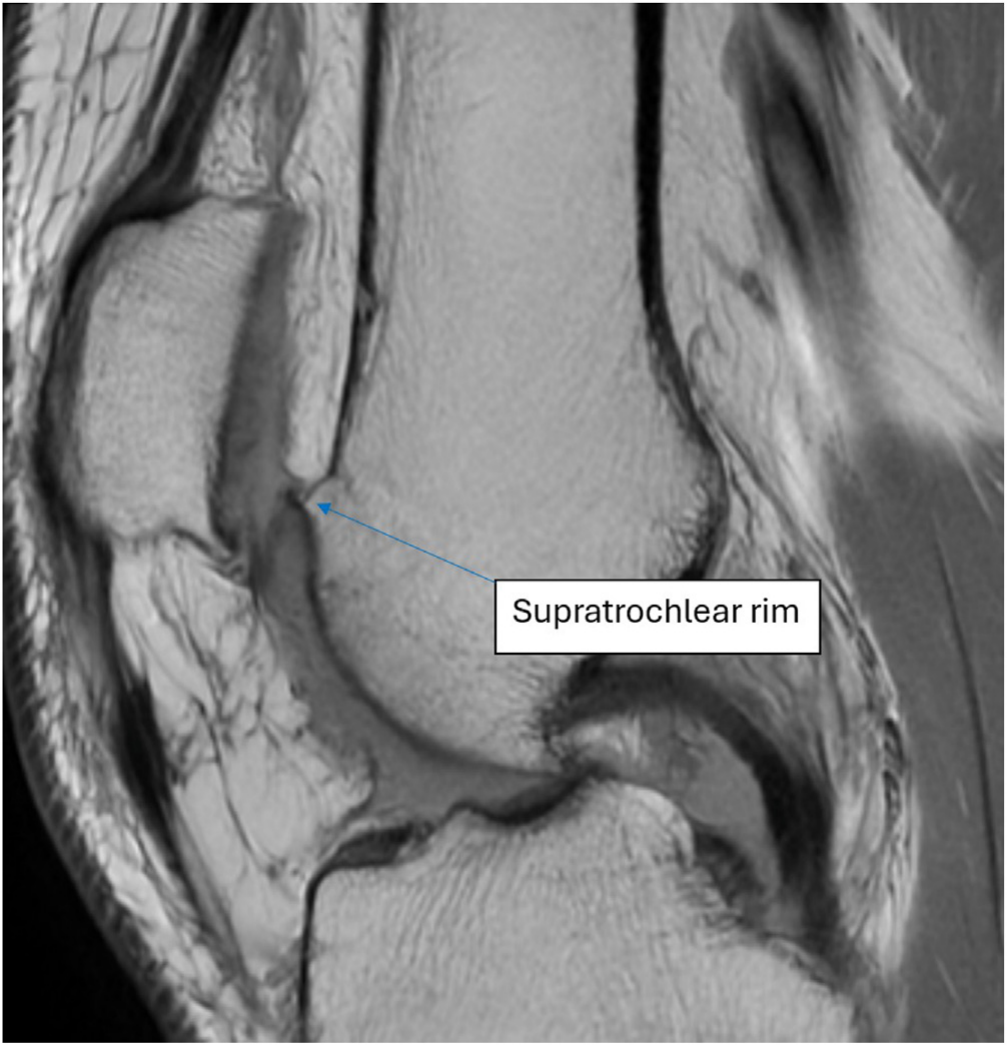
Supratrochlear rim on magnetic resonance imaging in sagittal view. The arrow is pointing to the bony supratrochlear rim. The presented cross section is the slice in which the anterior contour of the medial femoral condyle becomes visible, along with the rim. Magnetic resonance imaging diagnostics of the knee joint are required to confirm the presence of the rim before planning the surgical procedure. This imaging step also assesses the state of the patellar cartilage.
